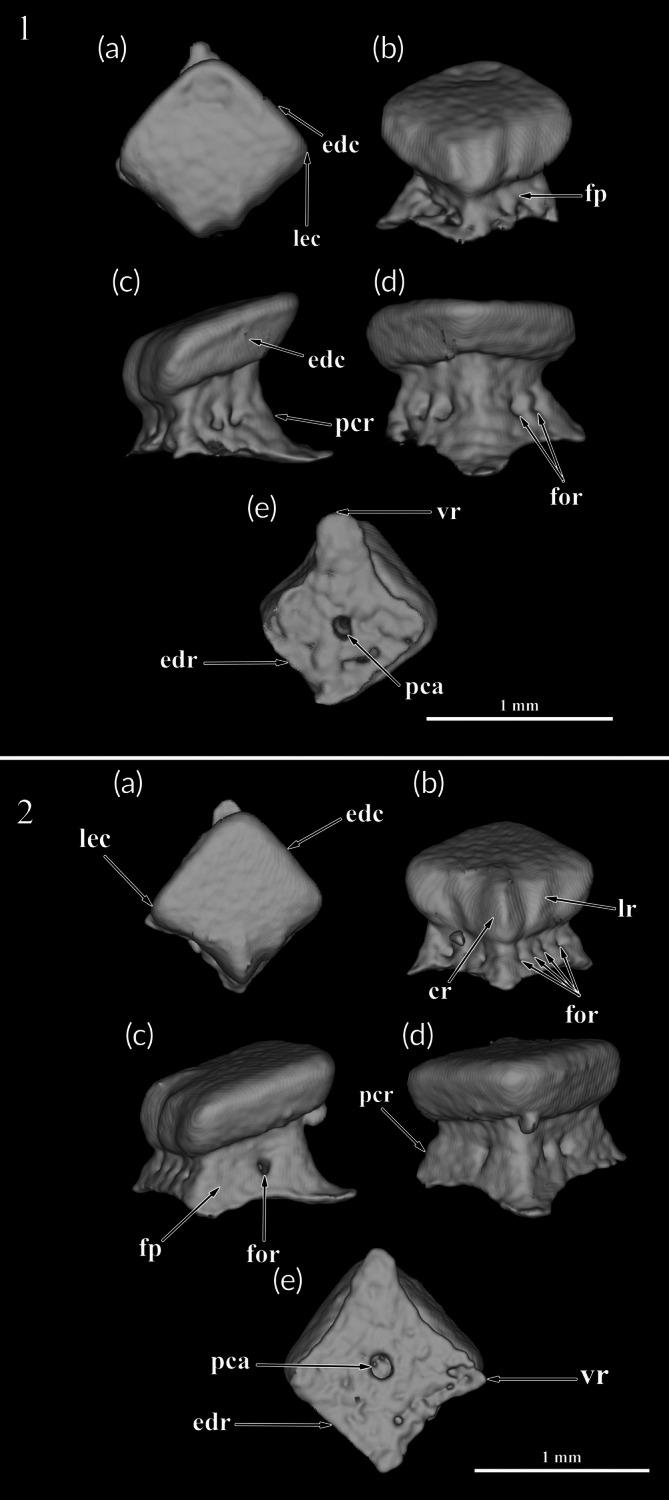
1 = Ventral type (V1 denticles; UFPB 14343 female, 1068 mm PCR). (1a) V1 dorsal view. (1b) V1 frontal view. (1c) V1 lateral view. (1d) V1 posterior view. (1e) V1 ventral view. In (1a, 1e) anterior to bottom; In (1b) anterior to front; In (1c) anterior to left; In (1d) anterior to back. 2 = Ventral type denticles (V2 denticles; UFPB 14343 female, 1068 mm PCR). (2a) V2 dorsal view. (2b) V2 frontal view. (2c) V2 lateral view. (2d) V2 posterior view. (2e) V2 ventral view. In (2a, 2e) anterior to bottom; In (2b) anterior to front; In (2c) anterior to left; In (2d) anterior to back (micro CT‐Scan).
